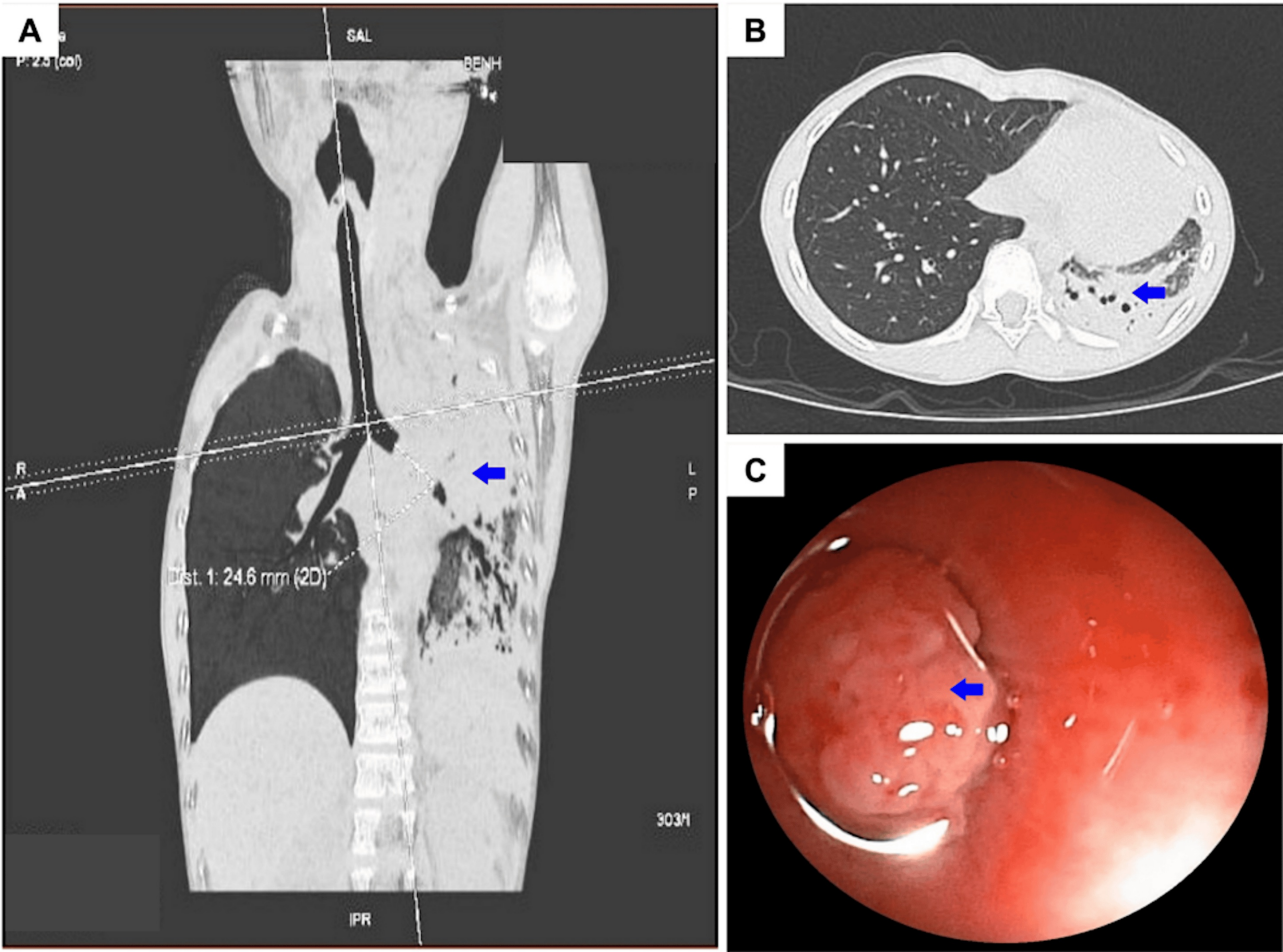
Computed tomography (CT) scan of the patient. (A) Endobronchial mass originating from the left main bronchus, causing significant compression of the left main bronchus and resulting in almost complete collapse of the left lung; (B) Left lung showing enlarged mediastinal lymph nodes, consolidation in the lower lobe, bronchiectasis, and evidence of air trapping; (C) Multifocal mass blocking the lumen of the left main bronchus as seen in bronchoscopy.
